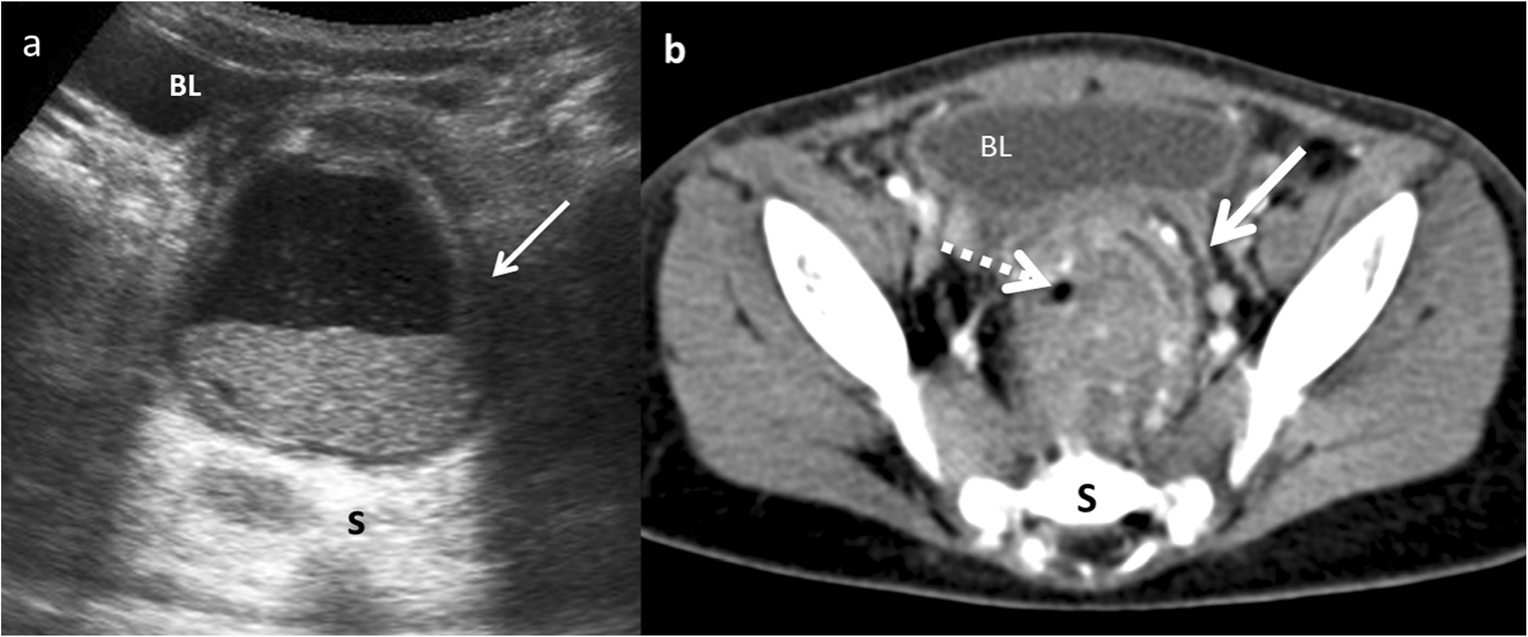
A 22-month-old boy with fever and abdominal pain. a Transversal US view of the pelvis shows a cystic mass between the bladder (*BL*) and the sacrum (*S*). The lesion presents anterior and left wall thickening (*arrow*) and contains a fluid-fluid level suggesting the presence of a complicated rectal mass. b Contrast-enhanced CT confirmed the presence of a complicated rectal cyst with remarkable inflammatory findings (*white arrow*) and gas (*dashed-line arrow*). Surgical findings: rectal duplication cyst with mucous and purulent content. Rectum muscular wall was oedematous and it was shared with the duplication cyst
